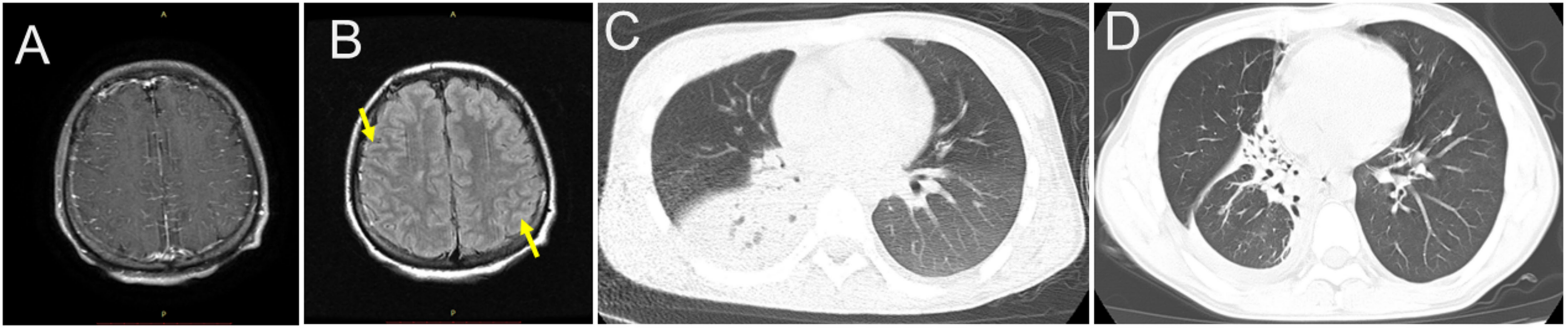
(A) Axial contrast-enhanced T1-weighted fat-suppressed MRI demonstrates thin, linear, homogeneous leptomeningeal enhancement along the sulci of the bilateral frontal, temporal, and parietal lobes, consistent with acute meningeal inflammation. No patchy meningeal thickening or abnormal dural enhancement is observed. (B) Axial post-contrast T2-FLAIR MRI shows multiple linear hyperintense signals within the cerebral sulci (arrows), suggesting inflammatory exudates in the subarachnoid space. No abnormal enhancement is noted in the cerebellar hemispheres or tentorium. (C) Axial chest CT showed consolidation and atelectasis in right middle and lower lobe. (D) Axial chest CT showed overall atelectasis in right middle lobe and partial atelectasis in right lower lobe.
